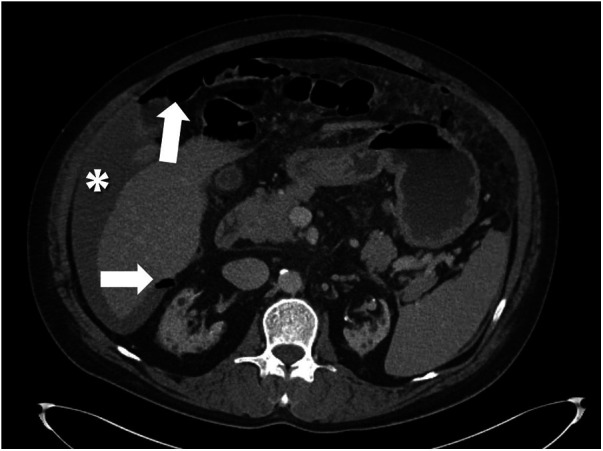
Abdominal CT findings suggestive of spontaneous bacterial peritonitis (SBP). The image reveals extensive pneumoperitoneum (arrow) and a large amount of free fluid collection (asterisk).
